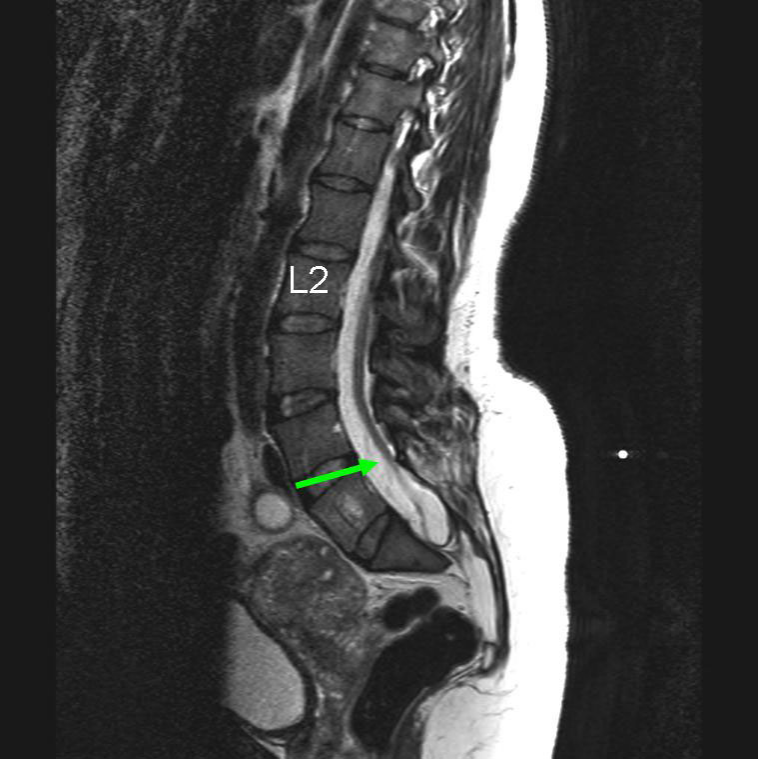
T2-weighted sagittal magnetic resonance imaging of the lower spinal cord. Green arrow points to elongated spinal cord.
